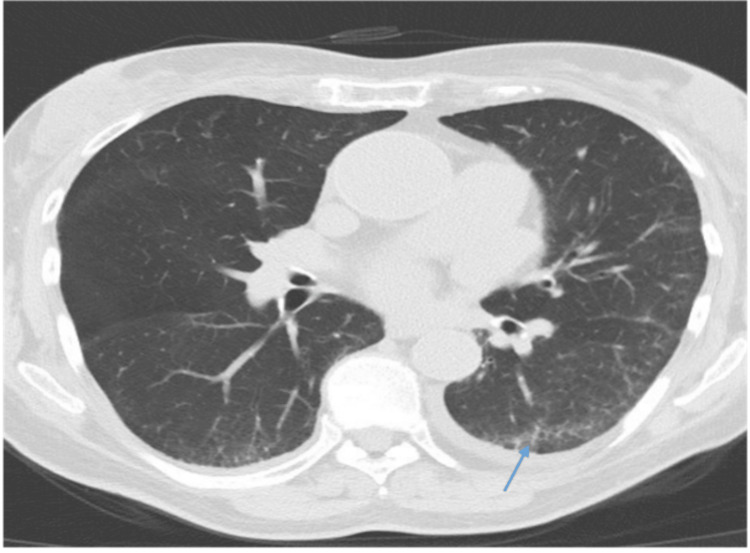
Follow-up axial high-resolution computed tomography (HRCT) chest image after three months of treatment demonstrating near-complete resolution of previously noted septal thickening and ground-glass opacities
